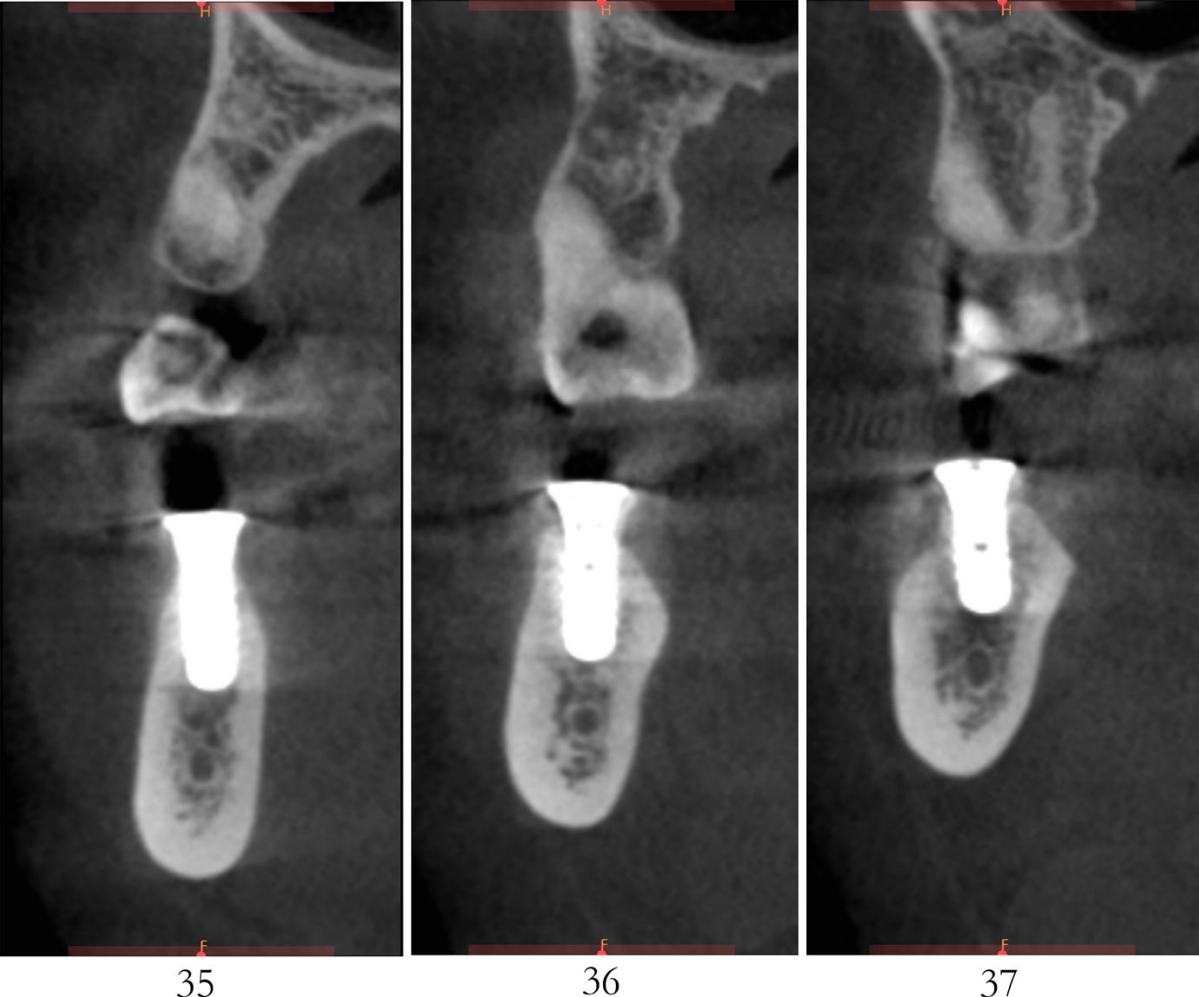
CBCT scanning the day before final restoration
